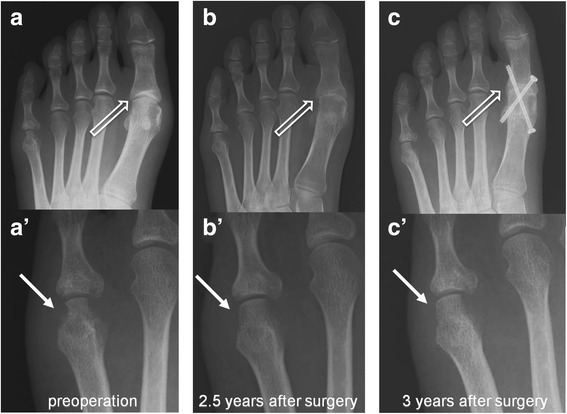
a–c, a′–c′ Bone regeneration after synovectomy of the 5th metatarsophalangeal joint of the left foot. The left panel shows the preoperative radiograph of the left foot. Bone erosion was seen in the 5th metatarsal head. The middle panel shows the reappearance of the 5th metatarsal head 2.5 years after synovectomy of the joint. Joint space narrowing appeared at the 1st metatarsophalangeal joint. The right panel shows consolidation of the 5th metatarsal head. The 1st metatarsophalangeal joint was fixed
